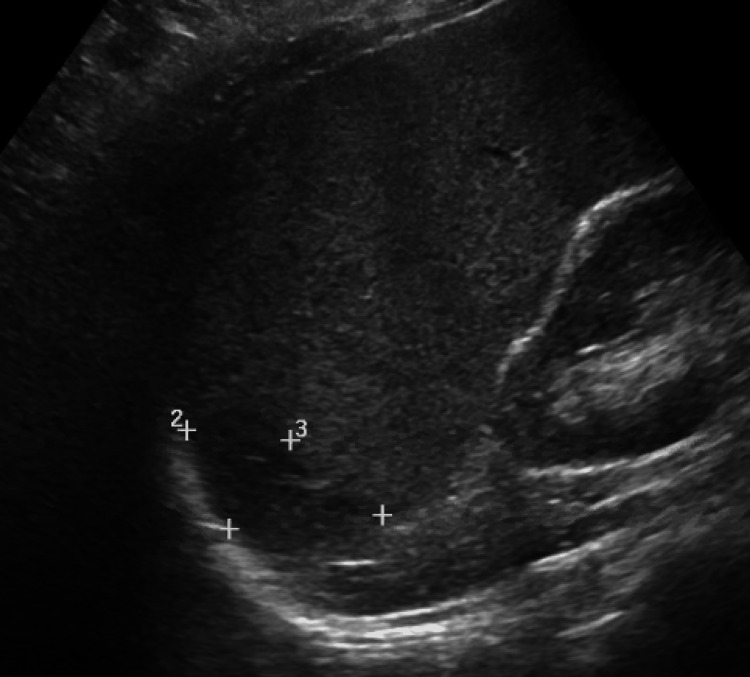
Abdominal ultrasound revealing splenomegaly and hypoechoic area measuring 5.4 cm × 3.7 cm × 3.7 cm.
